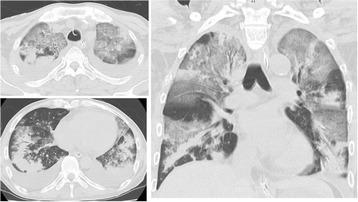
Computed tomography scans of the patient taken on the first ICU day. These images show the widespread bilateral peripheral field-dominant patchy consolidations accompanied with diffuse ground glass opacity.
